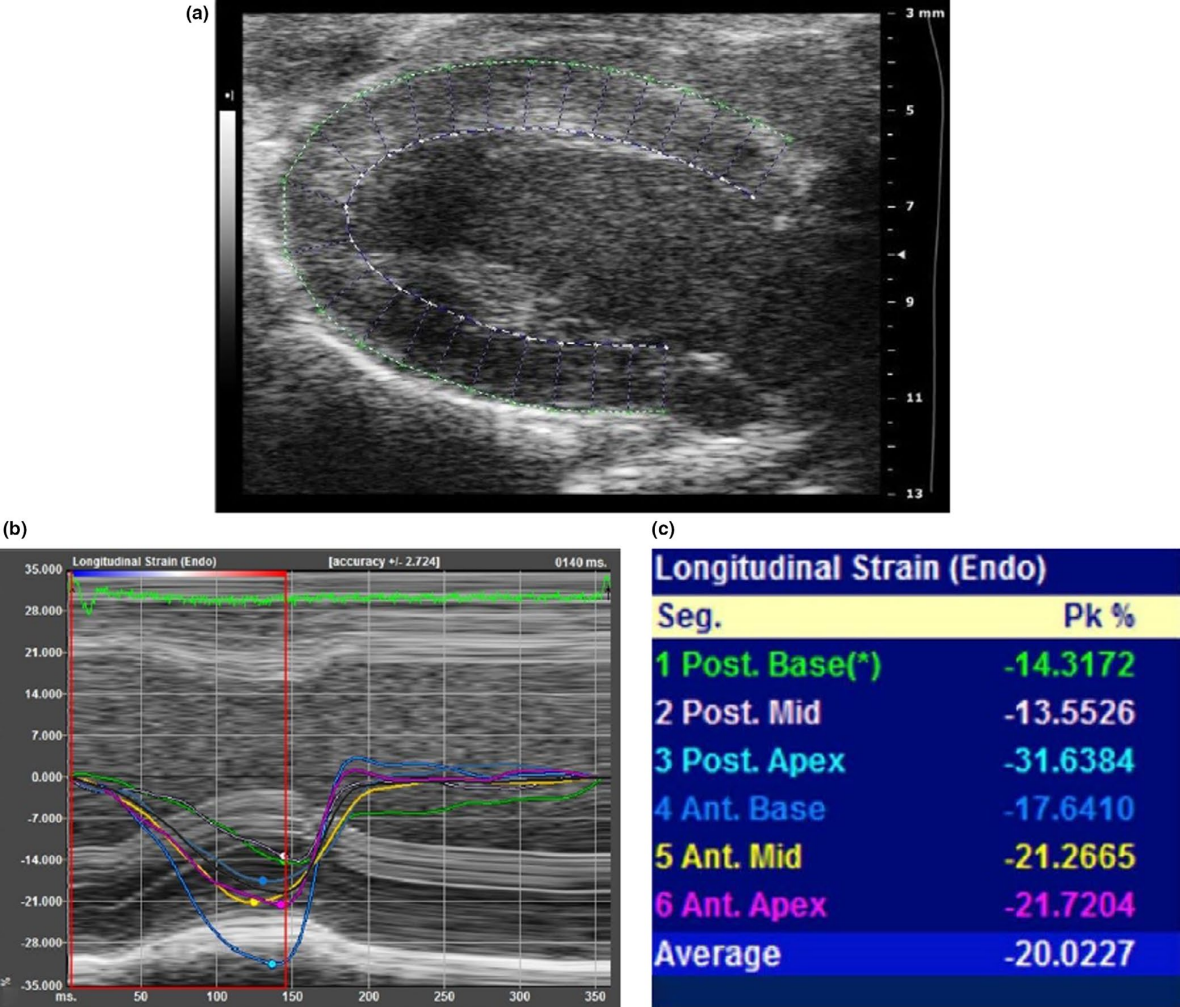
Myocardial strain analysis in hamsters from parasternal long‐axis view (PSLAX). (a) Analysis of endocardial (white dashed line) and epicardial (green outline) tracings; (b) longitudinal strain curves; (c) longitudinal peak systolic strain values and segmental nomenclature. Endocardial strain values are represented for global (white line = average of all segments), posterior basal (1); posterior mid (2); posterior apex (3); anterior basal (4); anterior mid (5); and anterior apex (6) segments
